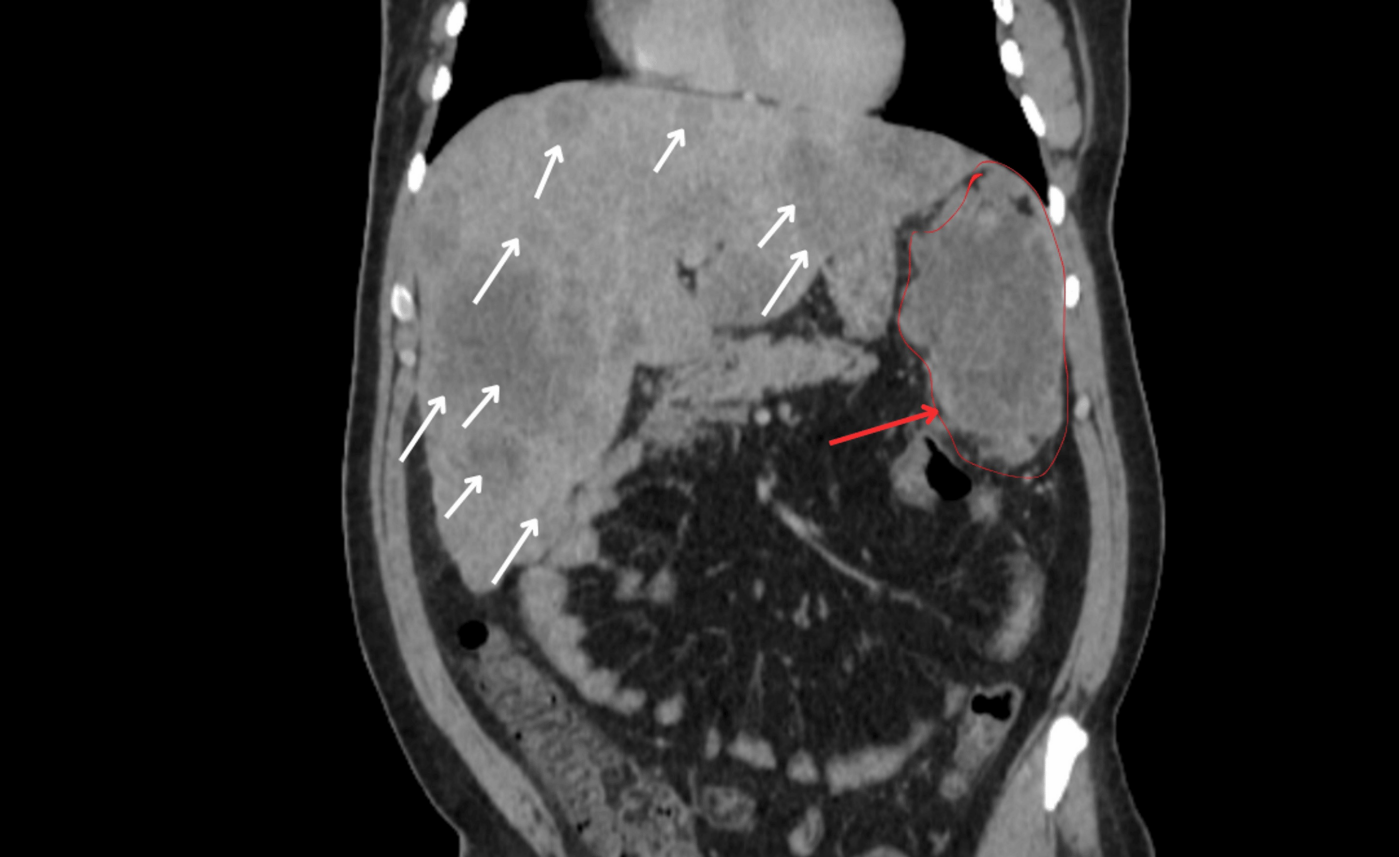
Coronal CT scan showing DSRCT peritoneal mass with hepatic involvement. DSRCT: desmoplastic small round cell tumor. Red arrow: Mass attached to the wall of the splenic flexure, measuring 113 × 61 mm. White arrows: Multiple peripheral, continuously enhancing lesions, consistent with liver metastases
